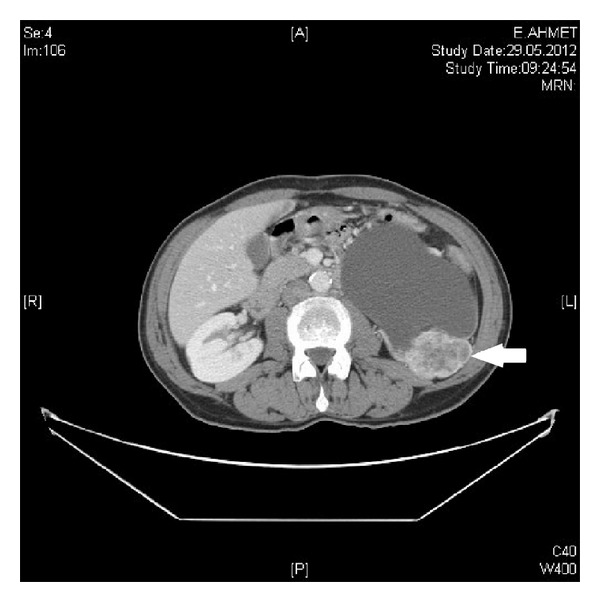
CT image of the solid renal parenchymal mass in the left kidney.
